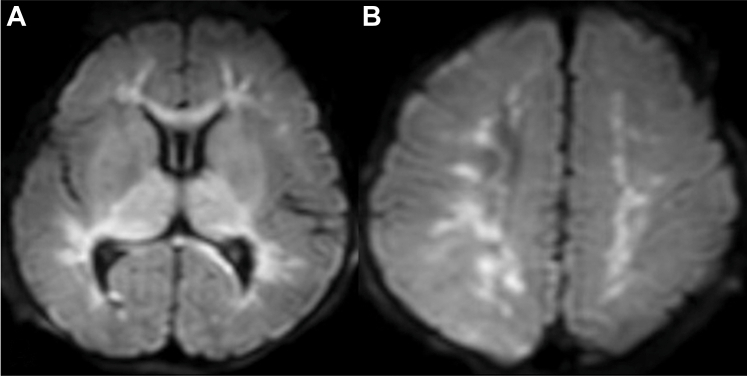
Preoperative Brain MRI in a 2-Day Old Infant (A and B) Axial diffusion weighted imaging showing injury to the subcortical, periventricular, and deep white matter, internal capsule, thalami and basal ganglia bilaterally consistent with hypoxic-ischemic brain injury. MRI = magnetic resonance imaging.
